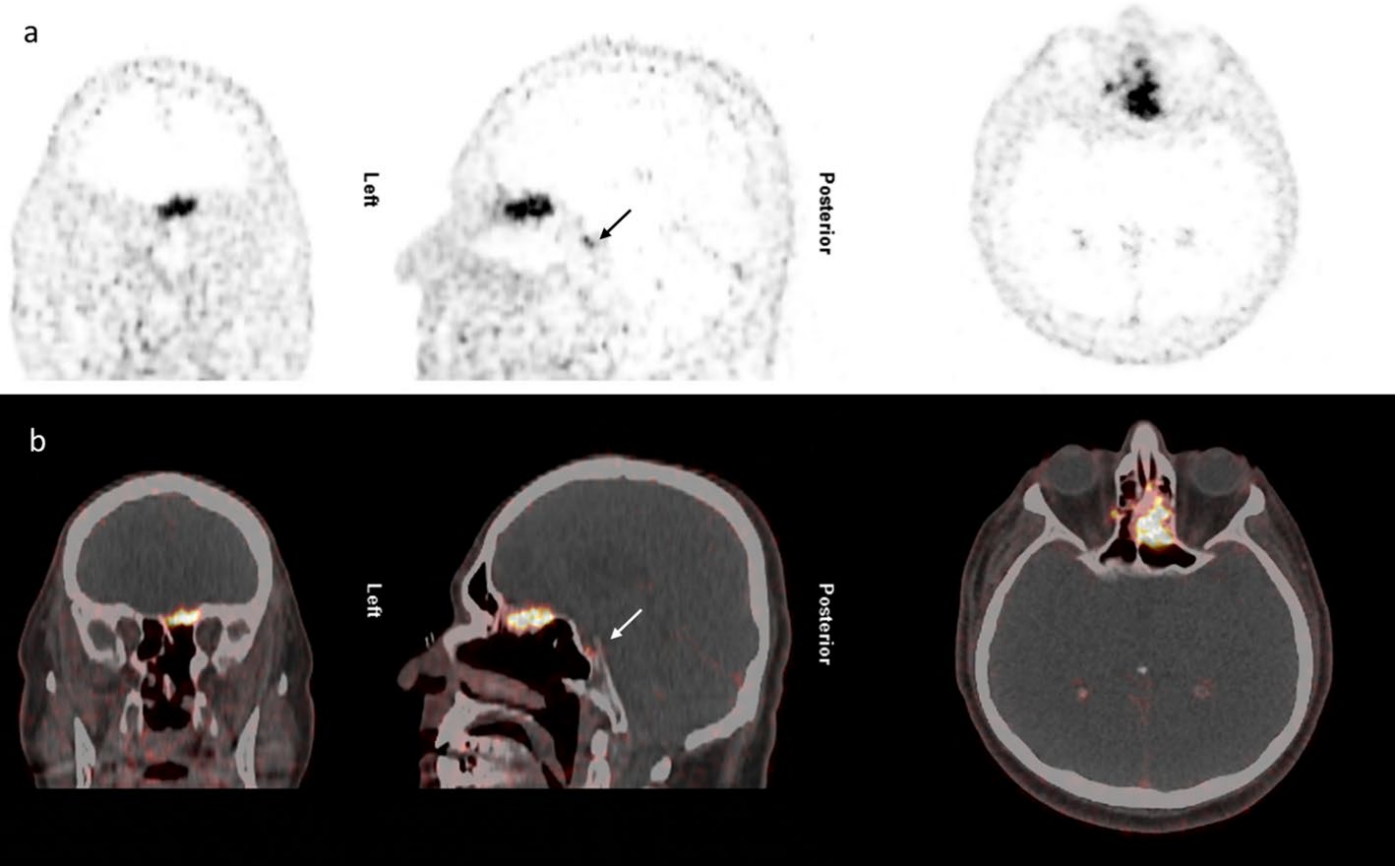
^68^Ga-DOTANOC PET/CT showing meningioma of the left olfactory nerve with intra- and extra-cranial tumor masses. Patient was a 64-year-old male neurosurgical treated before PET/CT. Stable disease and the WHO grade II allowed a “watch and wait” strategy. Arrow: physiological tracer uptake in the pituitary gland a: PET images and b: fused images in three planes
